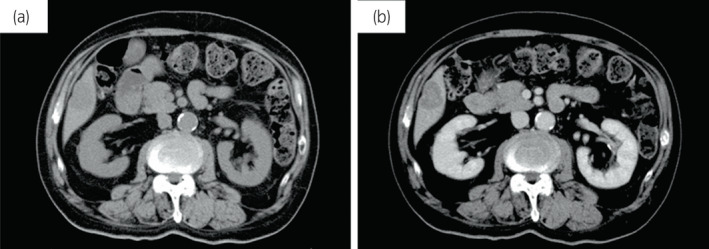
(a) Abdominal CT showed a solitary liver tumor with a diameter of 35 mm in the right posterior inferior segment of the liver. The tumor was not seen in previous scans. (b) Enhanced imaging revealed that the tumor had a concentric pattern with low density.
